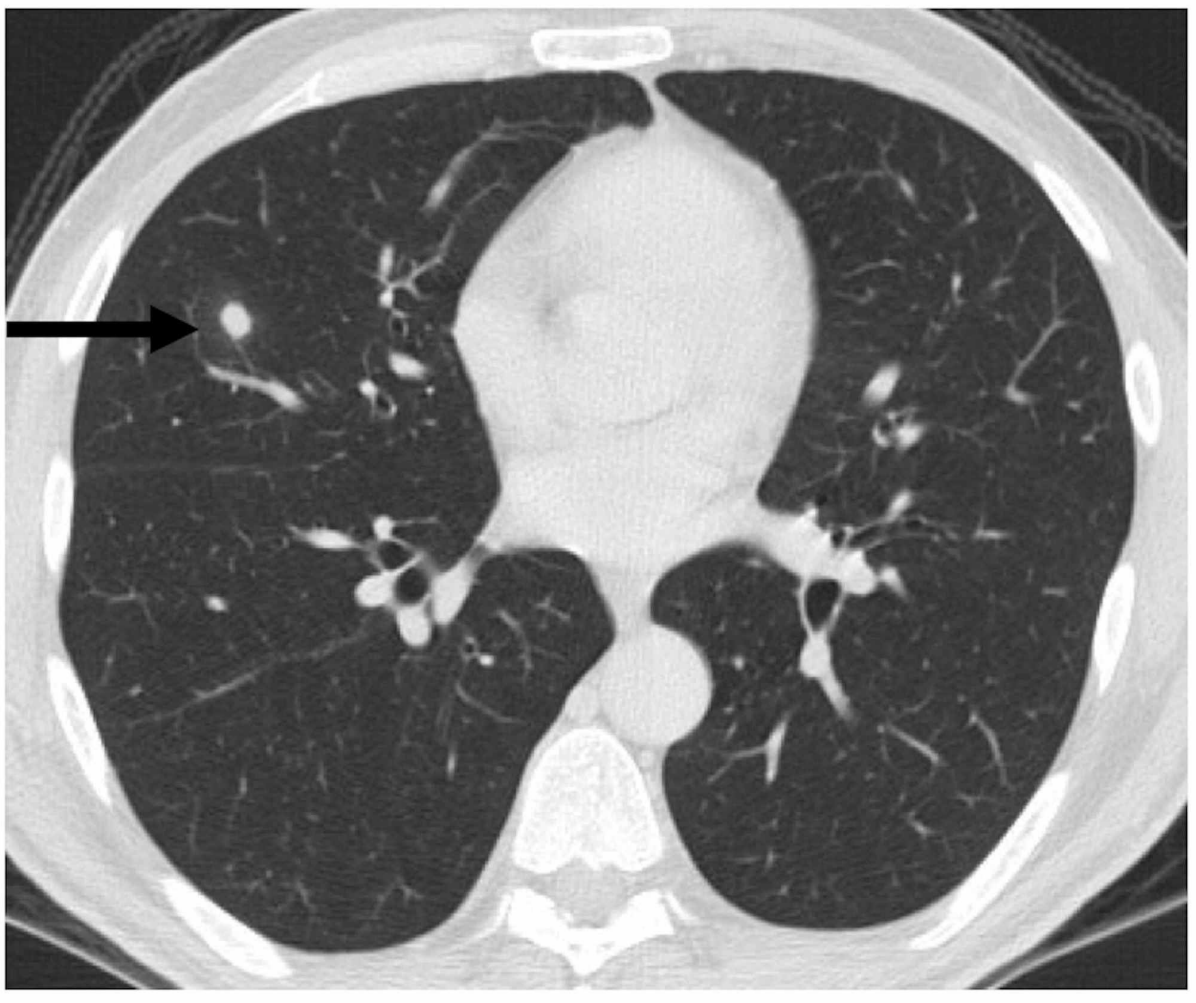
Enhanced axial thin-section chest CT (lung window) The image demonstrates a single right middle lobe round well-defined solid nodule (arrow), considered as newly developed pulmonary metastasis, i.e., disease progression CT: computed tomography
